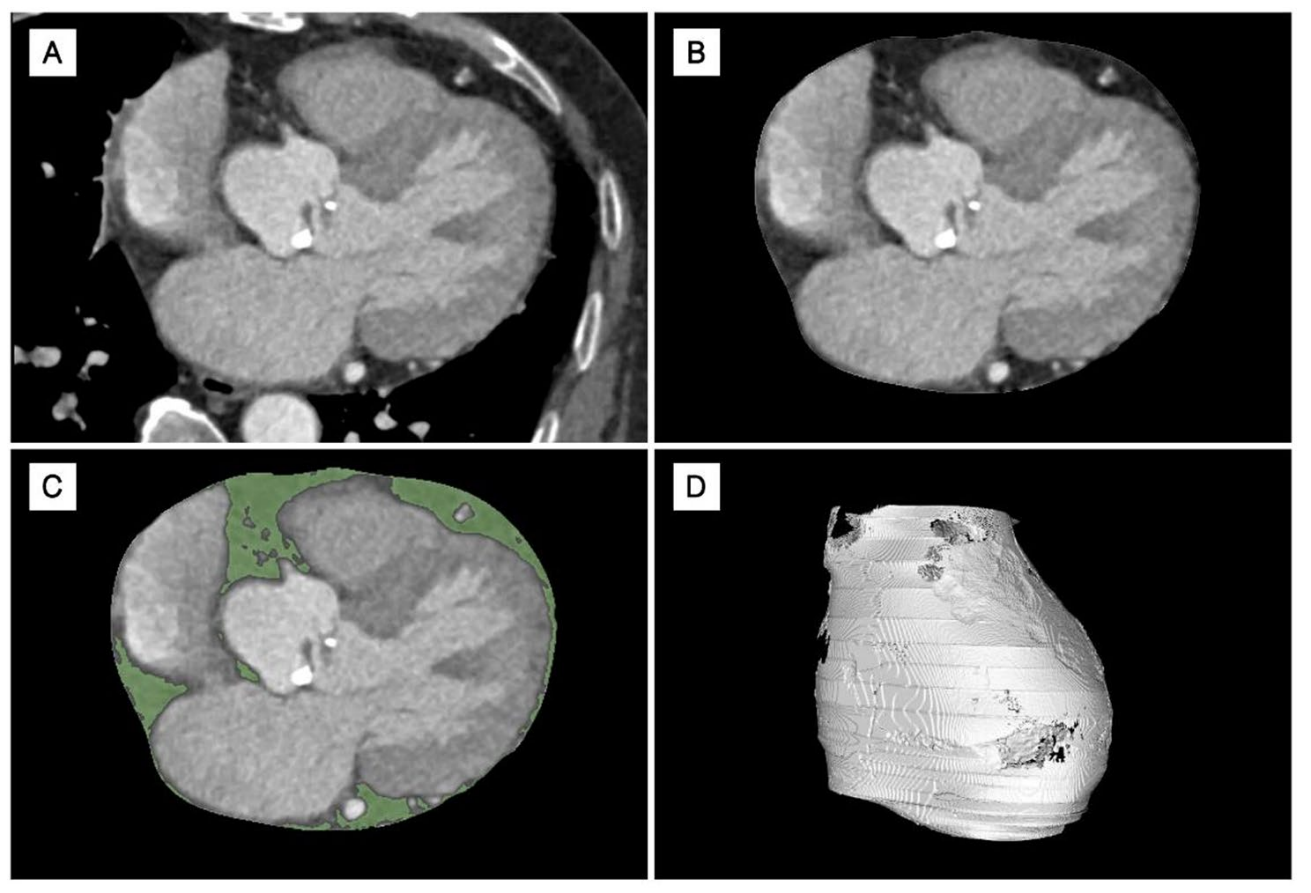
Measurement of EAT on CT imaging. Panel (A) manual contour of the pericardial border; Panel (B) automatic exclusion of structures outside the contour by the software; Panel (C) identification of the range of attenuation between − 10 and − 190 HU automatically by the software; Panel (D) The 3D reconstruction of the EAT layer and its volume was automatically obtained by multiplying the sum of all measured areas by the slice thickness
